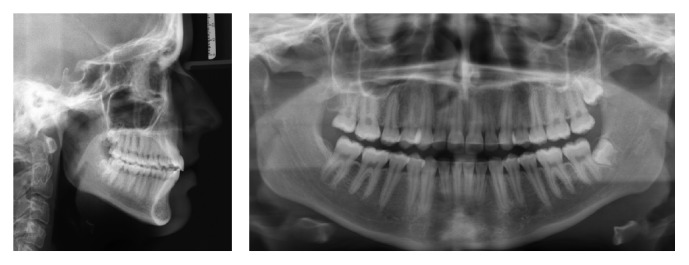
Four-year follow-up radiographs.
